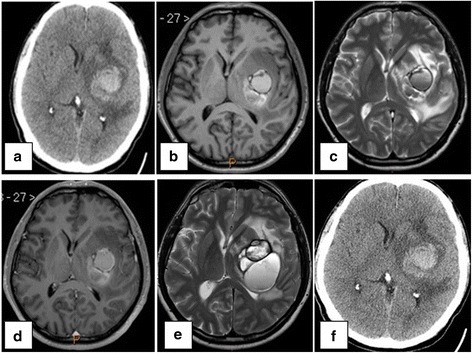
a Initial CT scan with left insular hematoma. b–d MRI-T1, T2, and T1 contrast, respectively, with multiloculate left insular hematoma, heterogeneous signal, and edema. e Follow-up MRI-T2 with new bleeding and higher mass effect. f Urgency CT scan with higher edema and mass effect
